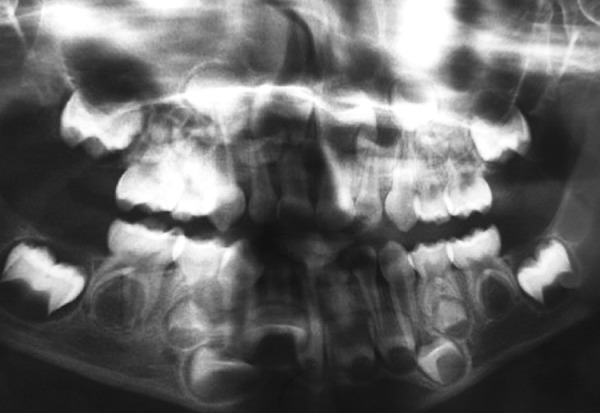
Panoramic radiograph. Note: Periapical radiolucency at tooth 85
